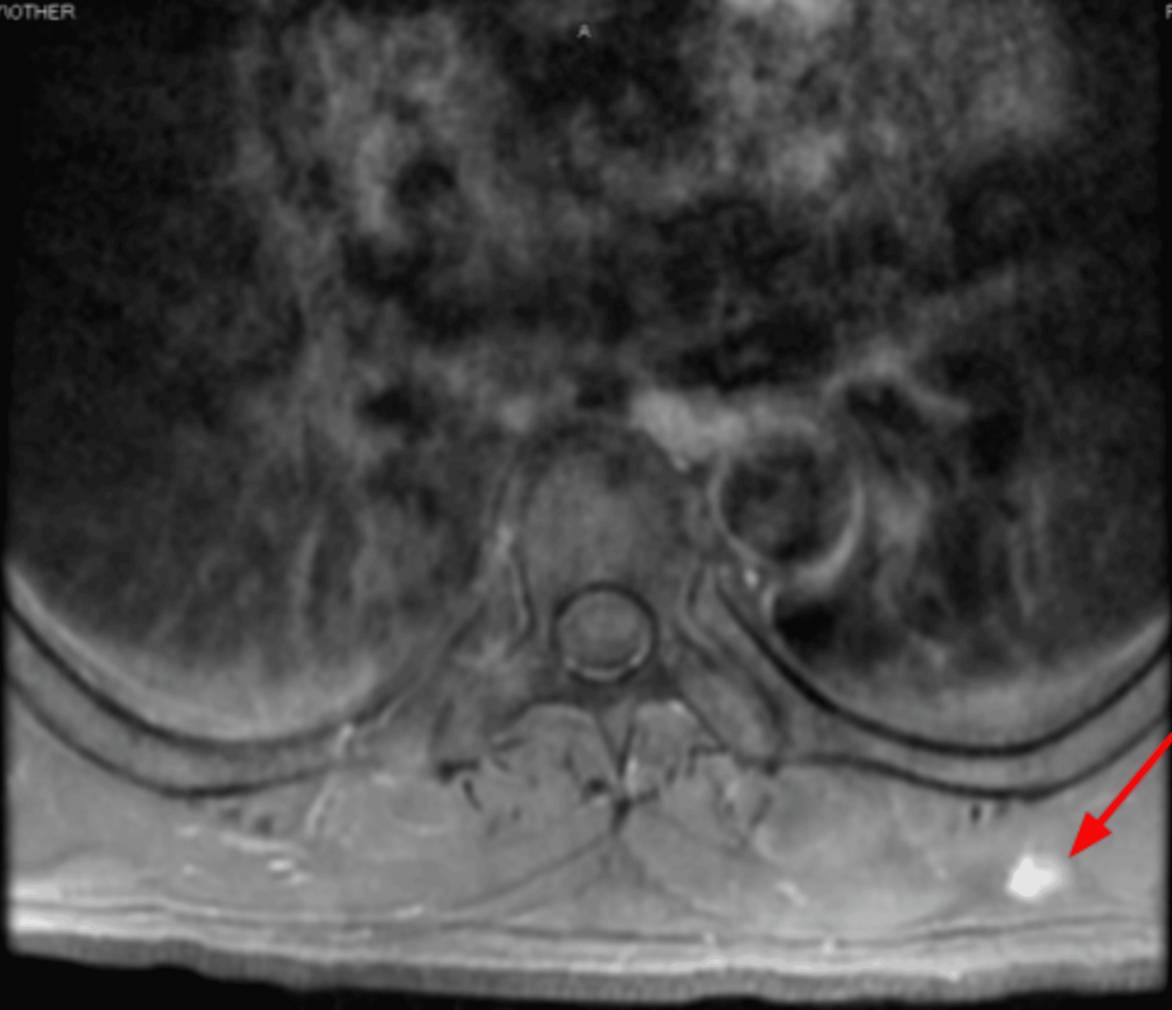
Chest CT (April 12, 2023) showing another enhancing hemorrhagic lesion in the soft tissue
